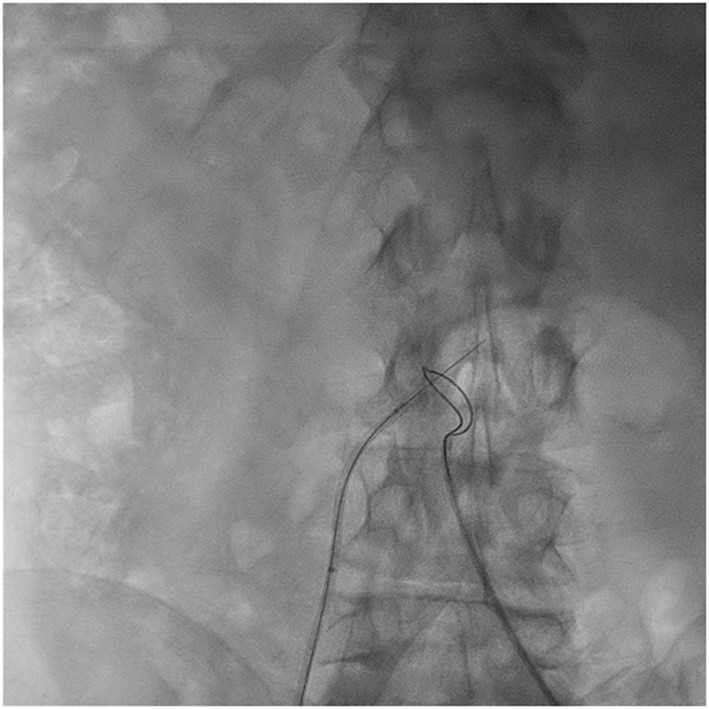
Caval to abdominal aorta traversal. A 0.014″ guidewire is positioned in the inferior vena cava pointing toward the abdominal aorta. A loop snare is positioned at the abdominal aorta at the level of the calcium-free window identified in preprocedural computed tomography. Using a catheter electrosurgery technique, the guidewire is electrified and advanced into the abdominal aorta to the center of the snare.
